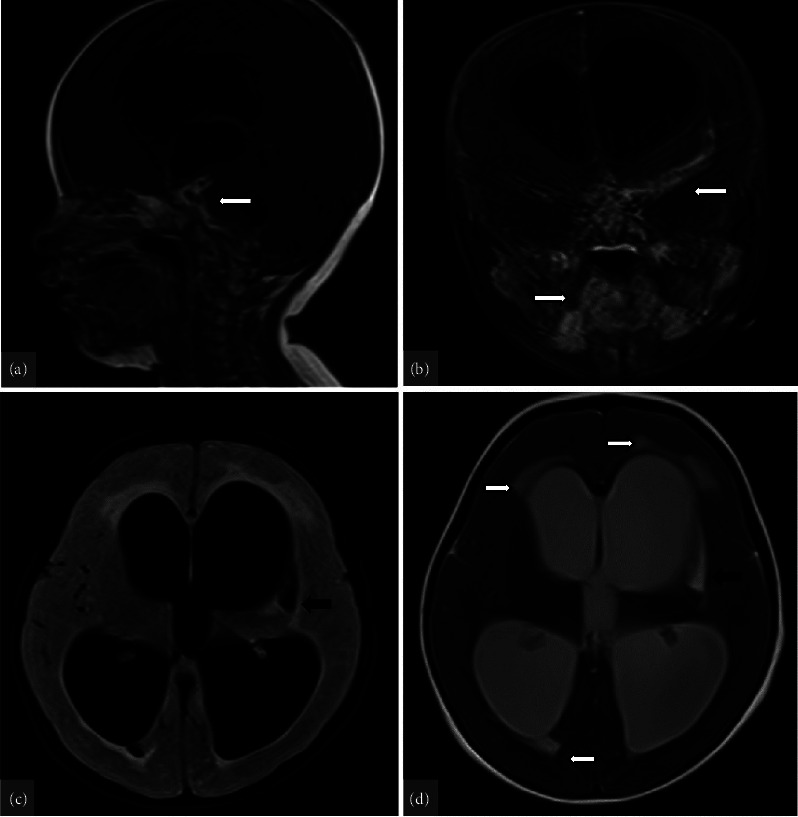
Brain MRI during the first admission. (a and b) T1 fat saturation with contrast showed intense nodular leptomeningeal enhancement in the basal, suprasellar, and prepontine cisterns and left Sylvian fissure. (c and d) FLAIR and T2W sequences showing a moderate hydrocephalus with asymmetrical lateral ventricle dilatation (right: 30 mm and left: 34 mm), leading to interstitial periventricular edema (white arrows). Black arrows show an old ischemic insult (encephalomalacia) of the left lentiform nucleus.
